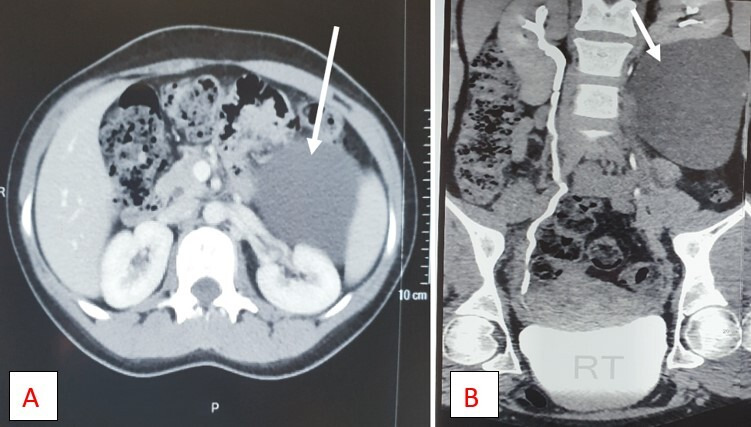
computed tomography scan showing: A) cystic lesion in left anterior pararenal space (arrow) in axial view; B) the lesion displacing the proximal and the midpart of the left ureter medially in coronal section
